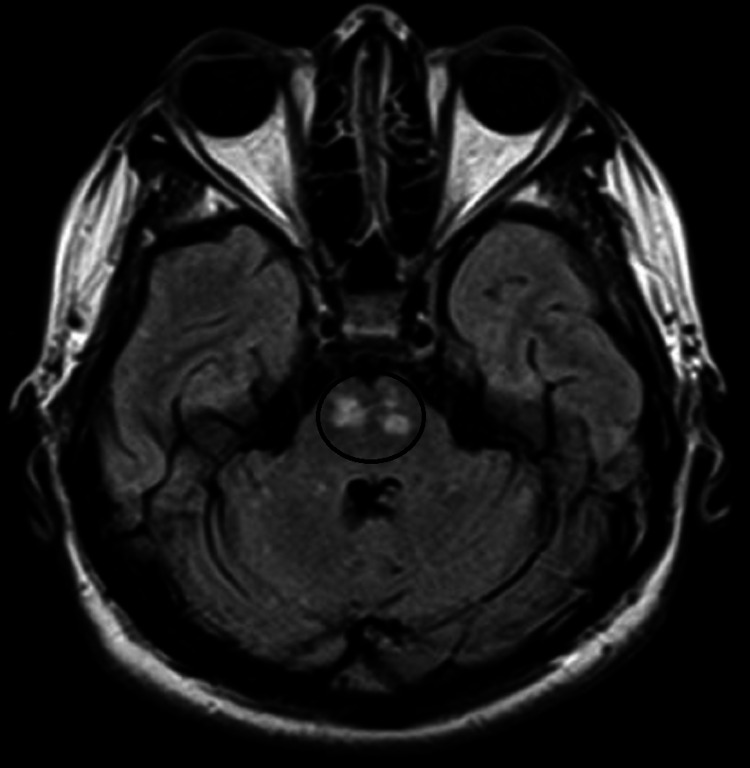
FLAIR axial MR image showing marked hyperintensity over bilateral pons (marked by a black circle) FLAIR: Fluid-attenuated inversion recovery
